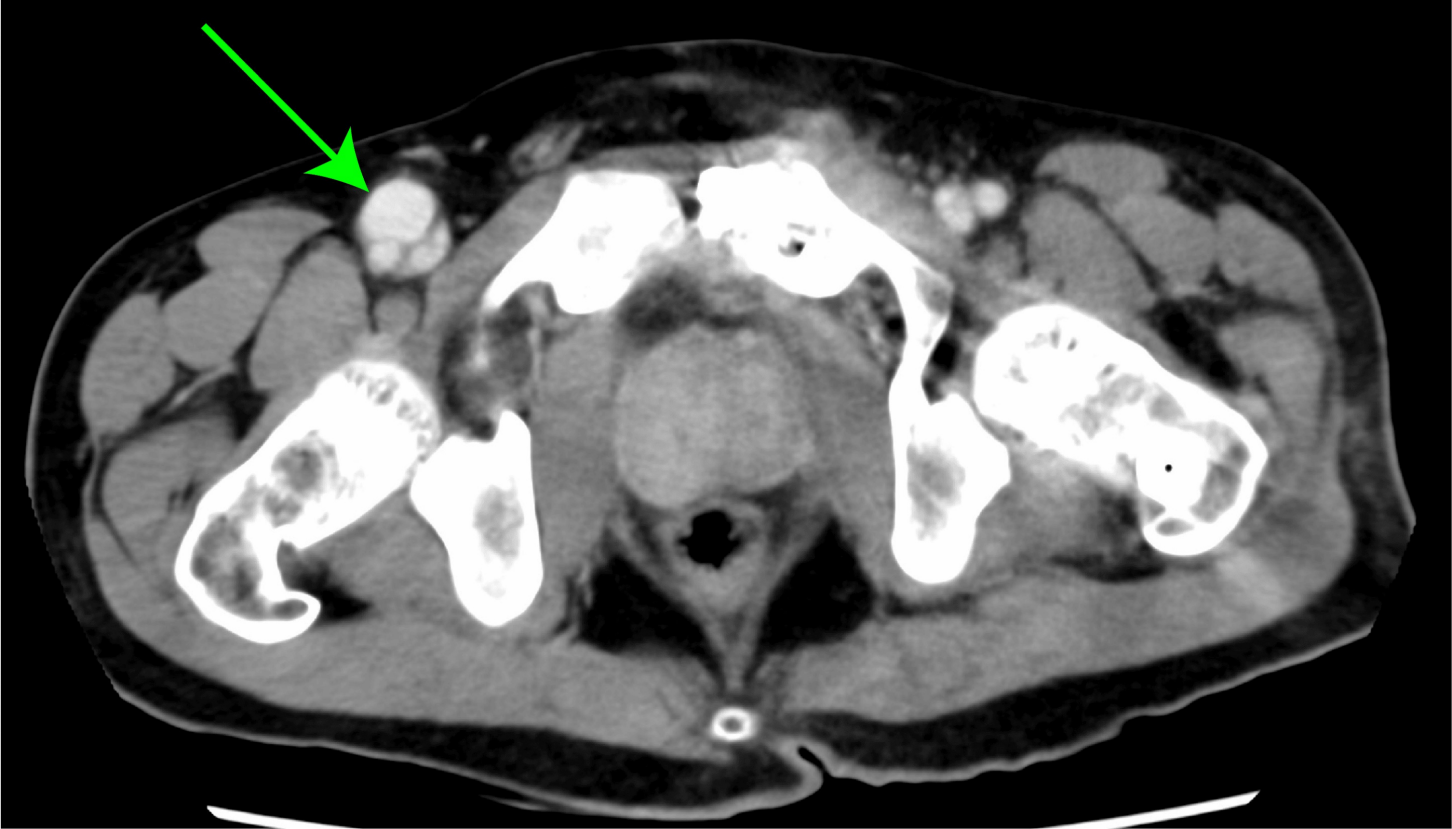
Imaging on postinjury day 14. Contrast-enhanced CT demonstrated the presence of a right femoral artery pseudoaneurysm (green arrow), which was enlarged compared with that in the previous scan. CT: computed tomography
